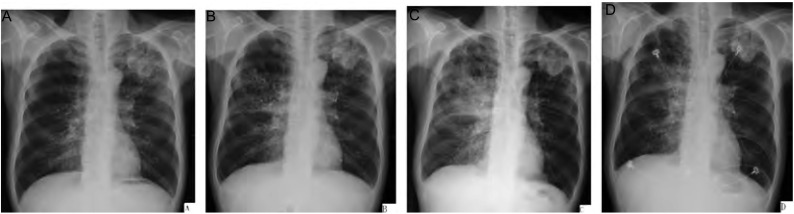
用吉非替尼前后及用激素前后胸片变化。A：吉非替尼治疗前，左上肺团块影；B和C：吉非替尼治疗后，右上肺渗出；D：糖皮质激素治疗后，右上肺渗出明显吸收。 Chest X-ray results comparison before and after gefitinib or glucocorticoids therapy. A: before gefitinib therapy; B and C: after gefitinib therapy; D: after glucocorticoids therapy.
